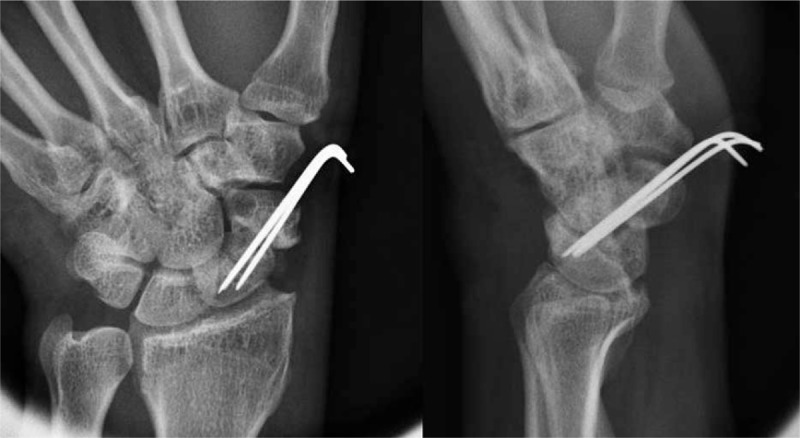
Immediate postoperative plain left wrist PA with ulnar deviation and lateral radiographs show internal fixation with K-wires and grafted bone at the nonunion site. PA = posteroanterior.
